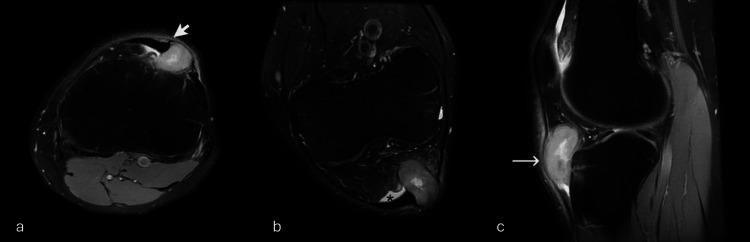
MRI images PD FS (a) axial, (b) coronal oblique, and (c) sagittal Showing soft tissue mass (long arrow) splaying the lateral part of the patellar tendon with claw sign (short arrow) with some edema in the adjacent infrapatellar fat tissue (asterisk).
